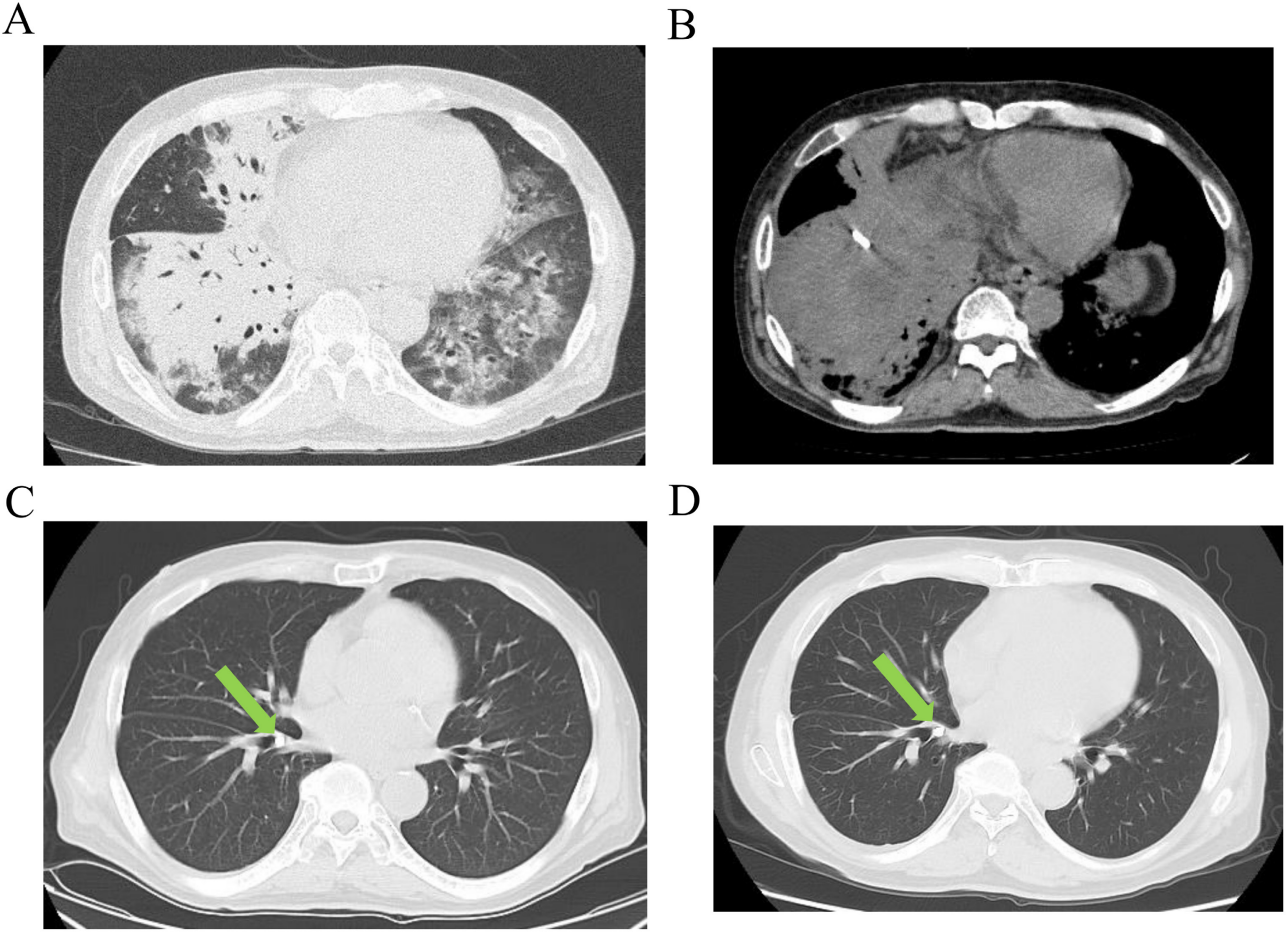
(A) Chest computed tomography (CT) upon admission showing bilateral pulmonary infiltrates, predominantly in the right lung. (B) Abdominal CT upon admission demonstrating an indwelling abdominal drain located immediately below the diaphragm. (C) Chest CT upon discharge revealing marked resolution of the pulmonary infiltrates. The Endobronchial Watanabe Spigot (EWS) is clearly visualised within the right B7 bronchus (arrow). (D) Chest CT at 6‐month follow‐up showing no recurrence of pneumonia. The EWS remains securely in place in the right B7 bronchus (arrow).
